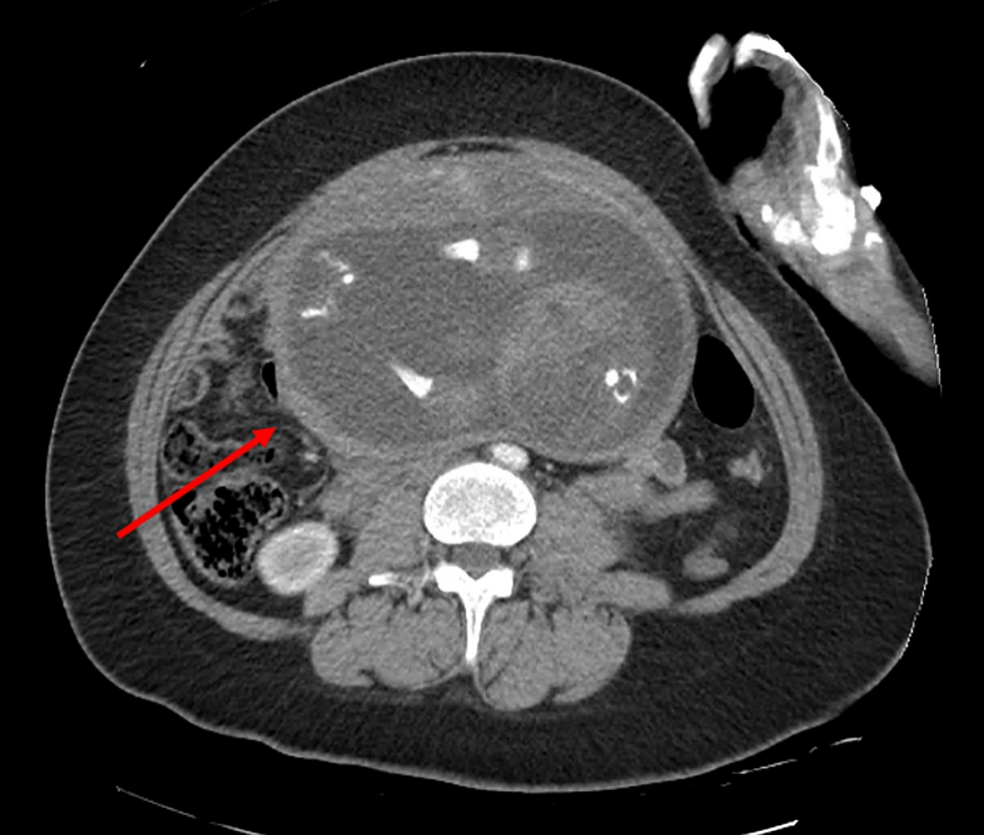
CT scan showing a small abdominal hematoma (arrow)
